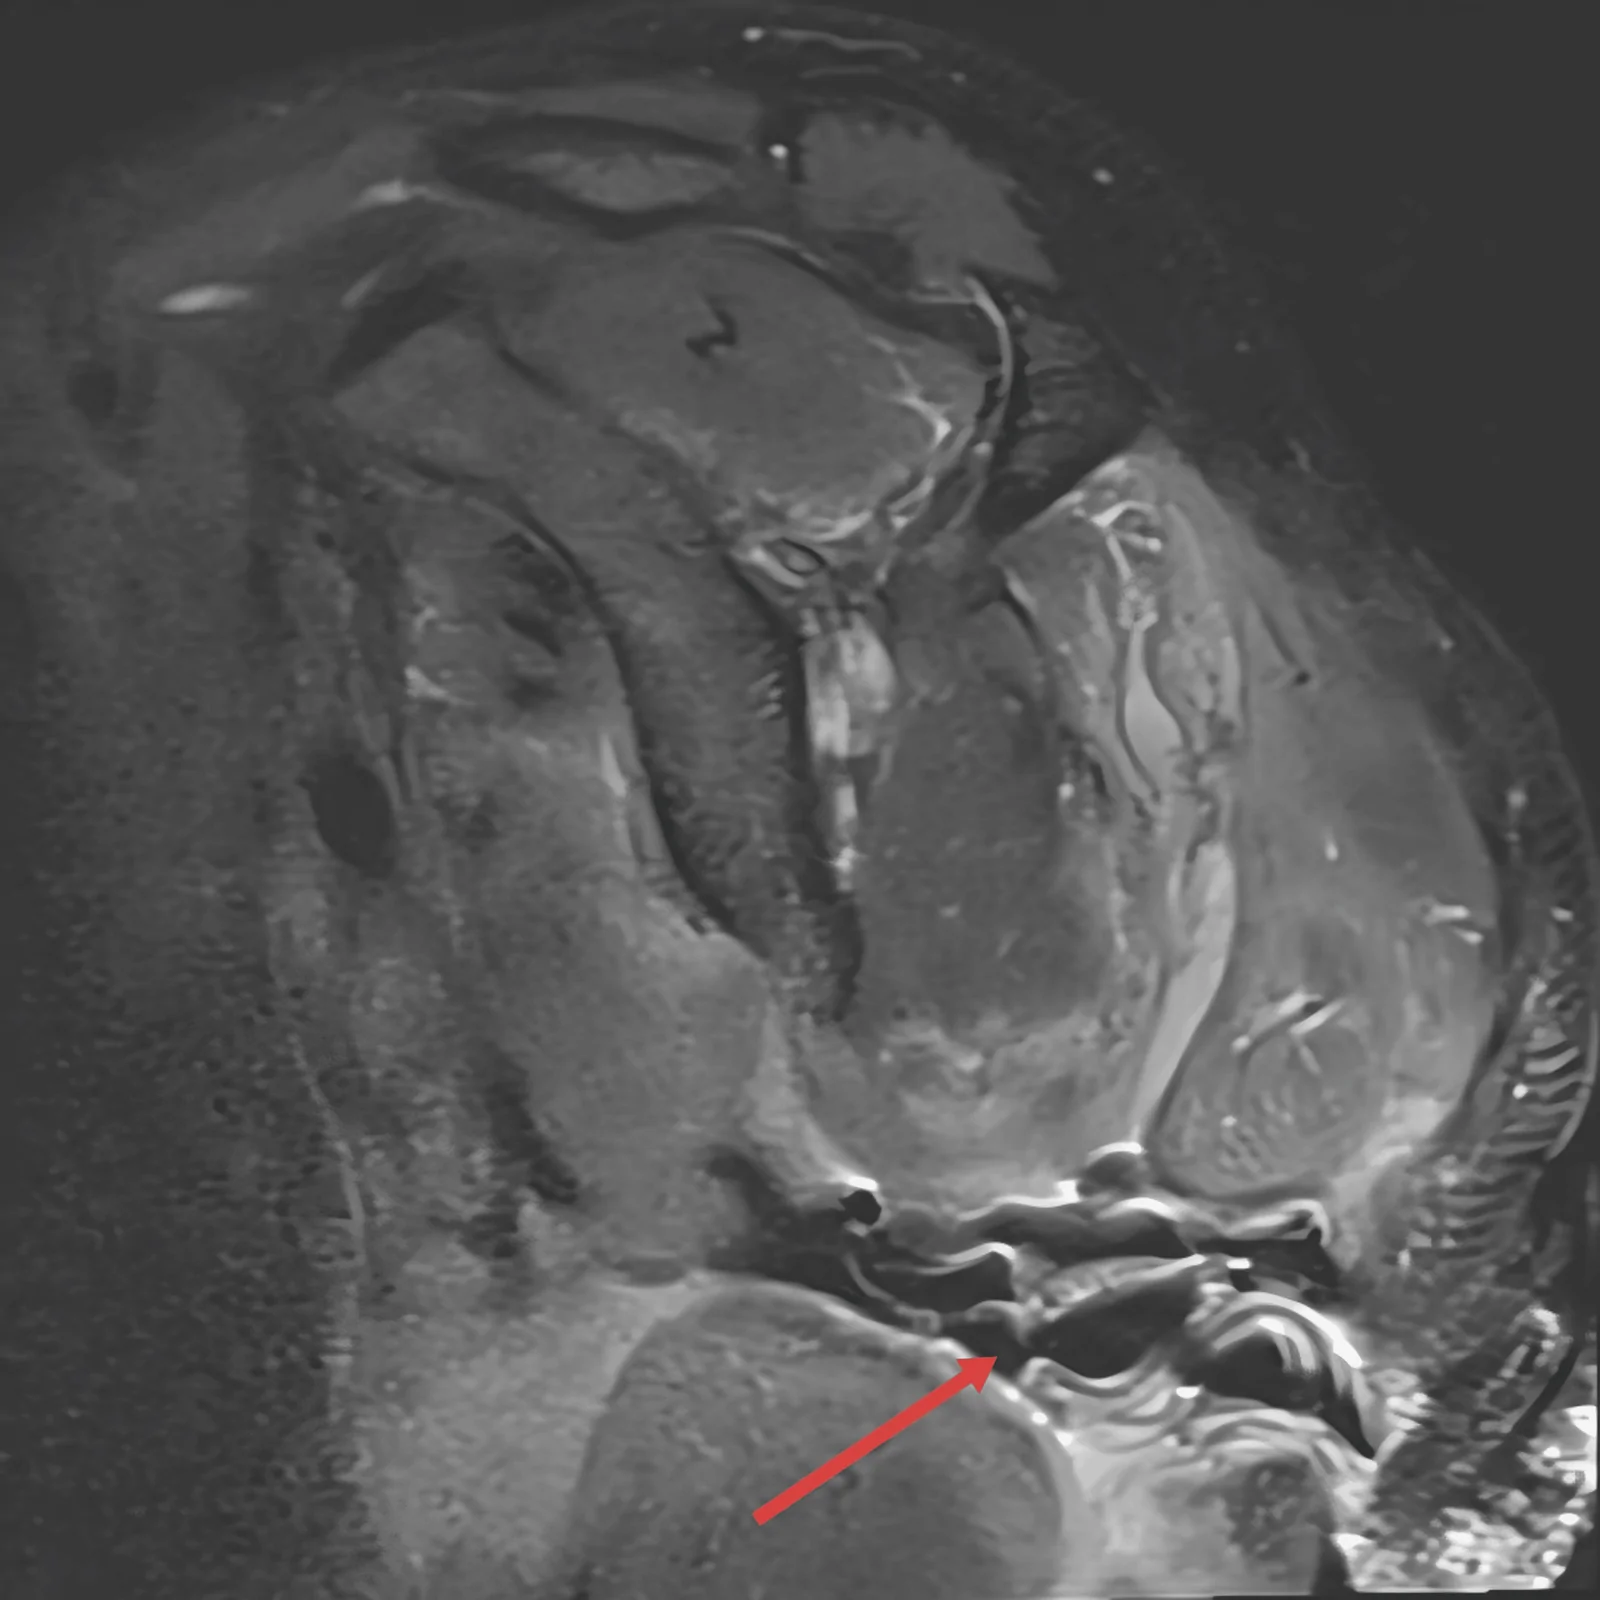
Repeat MRI of the right shoulder on postoperative day 12 from initial surgery, demonstrating a large surgical cavity (arrow) within the quadrilateral space between the teres minor and teres major muscles with adjacent reactive myositis in the T2 axial view MRI: magnetic resonance imaging
